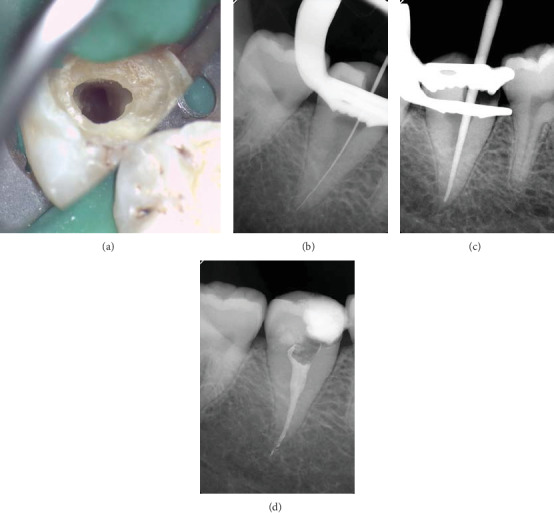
(a) Accessed cavity preparation shows the presence of one large root canal located in the center of the pulp chamber. (b) Working length determination radiograph. (c) Master gutta-percha cone radiograph. (d) Final radiograph showing dense obturation with sealer puffs.
